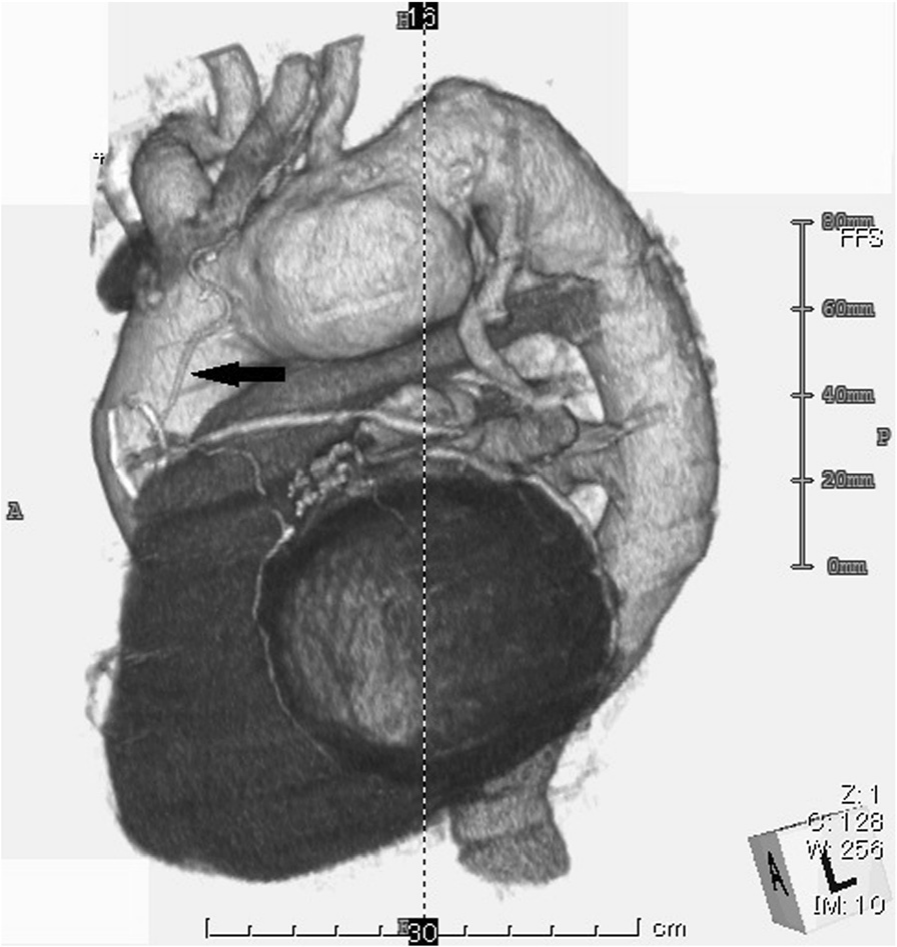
Preoperative computed tomography (CT) scan revealed saccular type aortic arch aneurysm and left internal thoracic artery bypass graft in left anterior descending artery as indicated by arrow.
